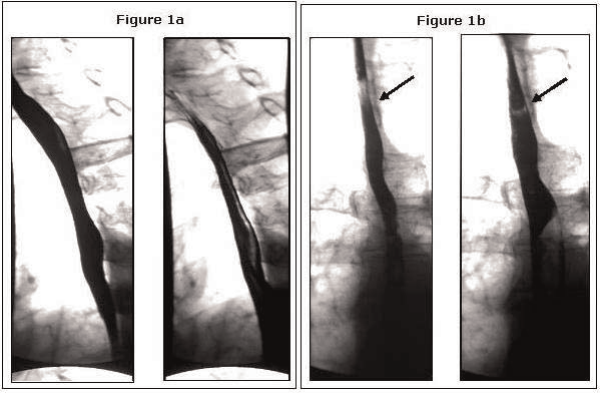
(A) Barium Swallow Radiograph - Liquid medium flowing freely. (B) Barium Swallow Radiograph - Solid medium (bread soaked in barium) is been held up in lower oesophagus.
